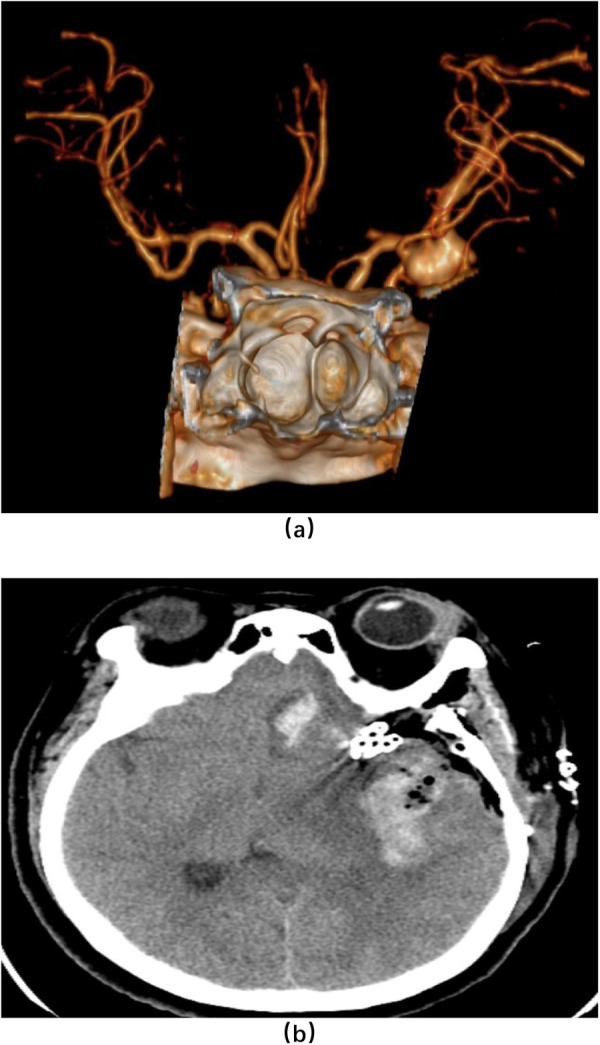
Imaging of a patient treated with microsurgical clipping (male, 65 years old). (a) shows preoperative head DSA; (b) shows postoperative three-dimensional CT of the head.
